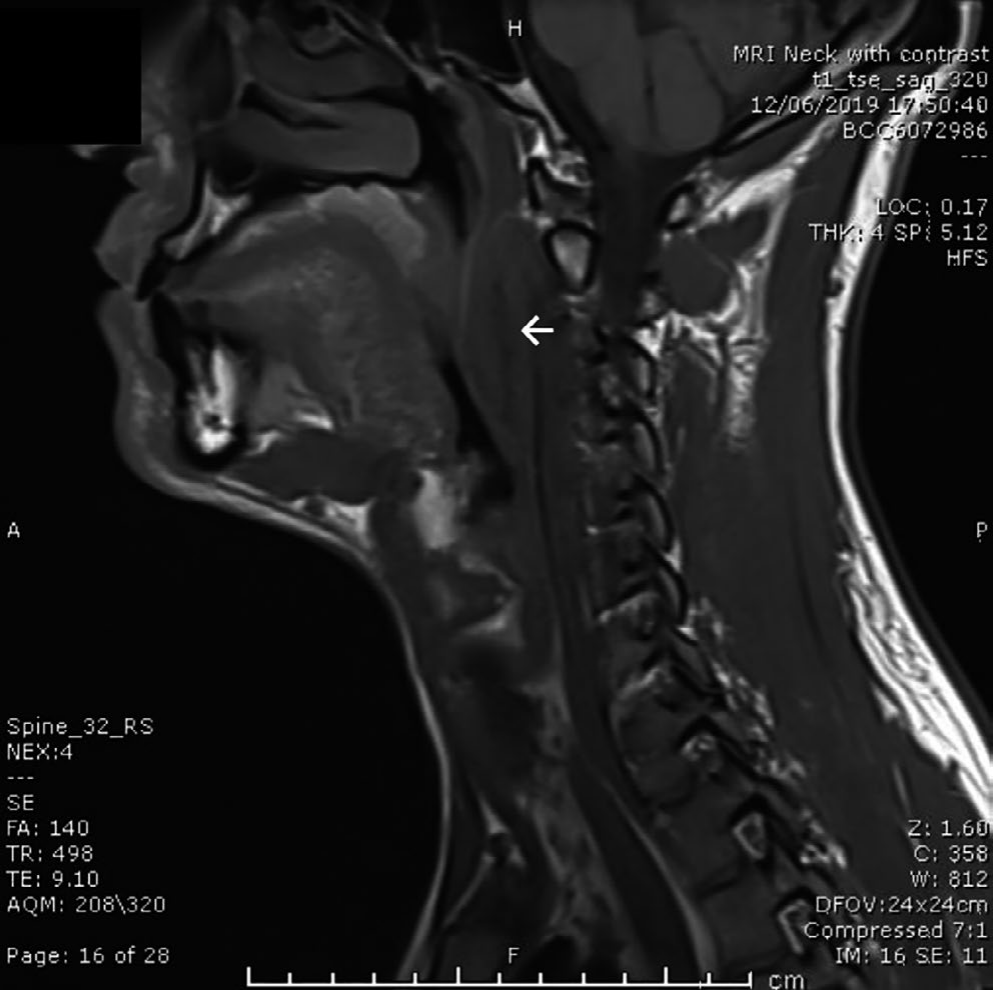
Coronal MRI section showing T1 image contrast‐enhanced
